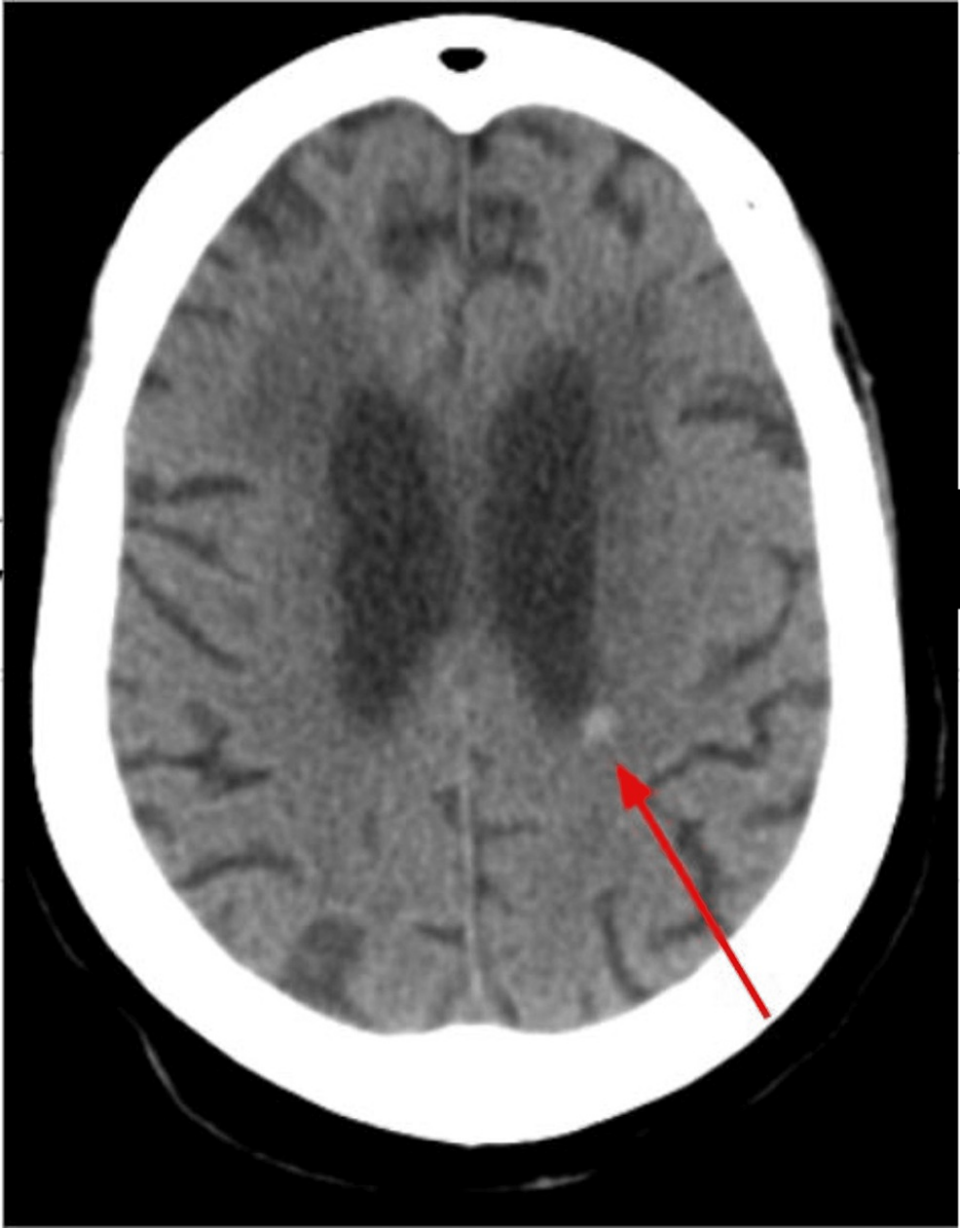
CT head without contrast showing small hyper-dense focus at the left centrum semiovale, suggestive of a small hyper-dense mass or subacute hemorrhage
